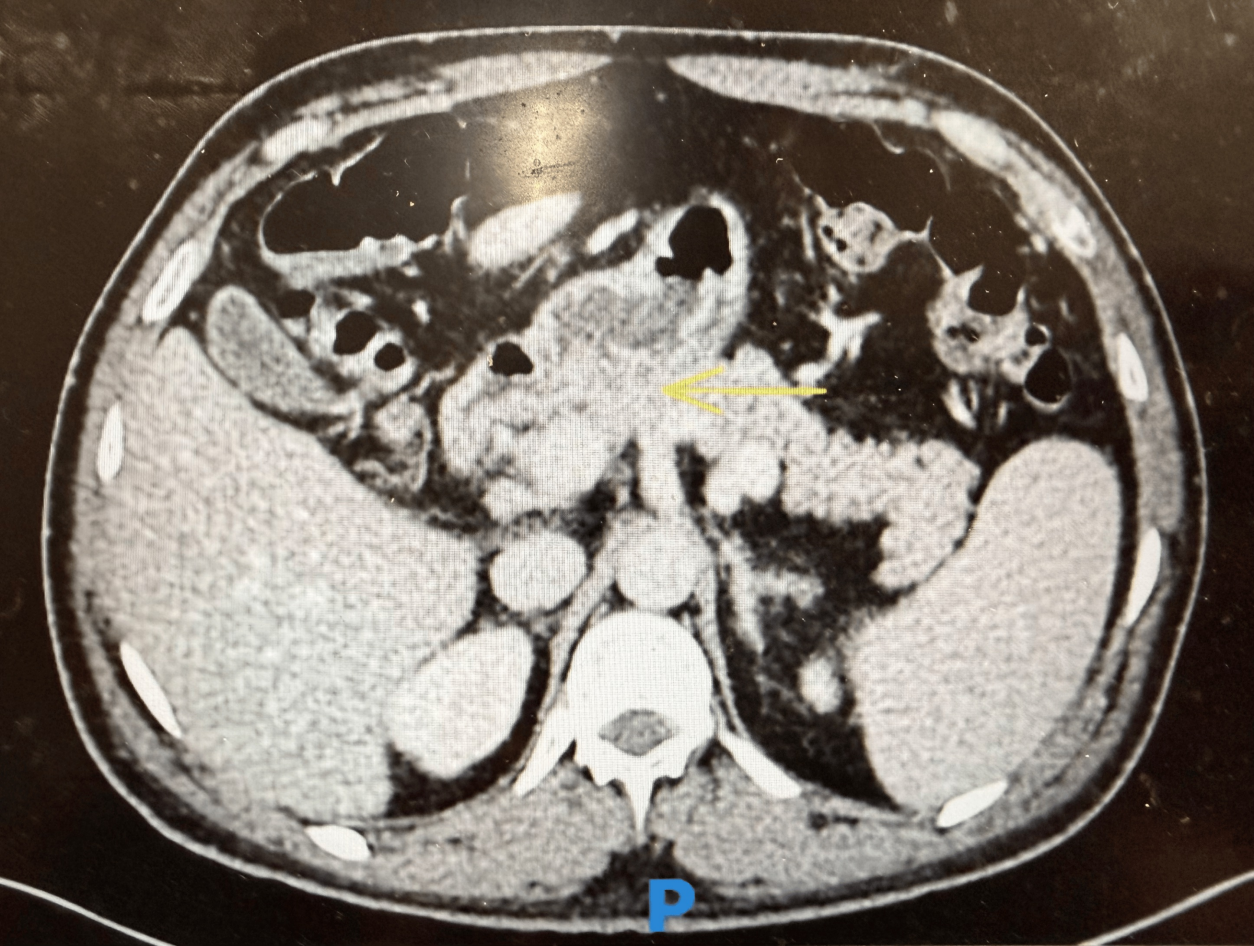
Contrast-enhanced CT of the abdomen showing duodenal fat stranding (yellow arrow).
